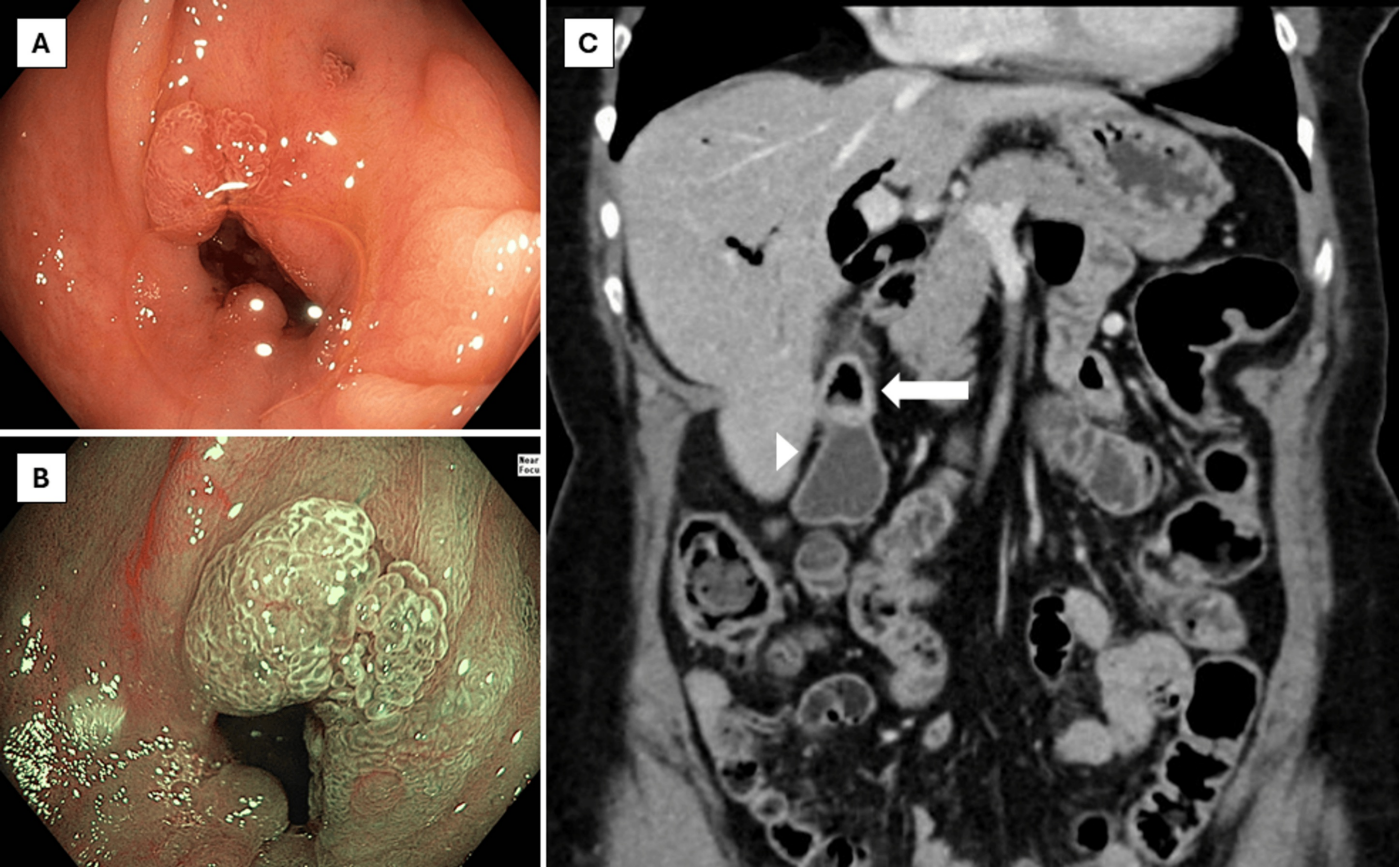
Initial colonoscopy and CT Panel A: White light endoscopy showing a diverticulum-like defect in the ascending colon. Panel B: Virtual chromoendoscopy (narrow band imaging, Olympus) of the defect. Panel C: Computed tomography (CT) with intravenous contrast (coronal view) showing close contact between gallbladder (arrow) and ascending colon (arrowhead) with pneumobilia.
